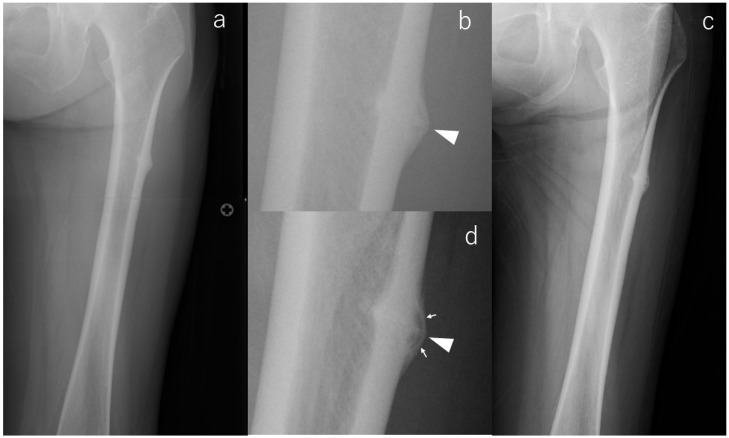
X-rays of case #2 at various time points. (a): Radiograph at the time of injury, (b): Enlarged image of a; radiolucency running across the cortex (arrowhead), (c): Radiograph at the last observation, (d): Enlarged image of (c); magnified fracture line (arrowhead) and radiolucency in the beak (arrows).
